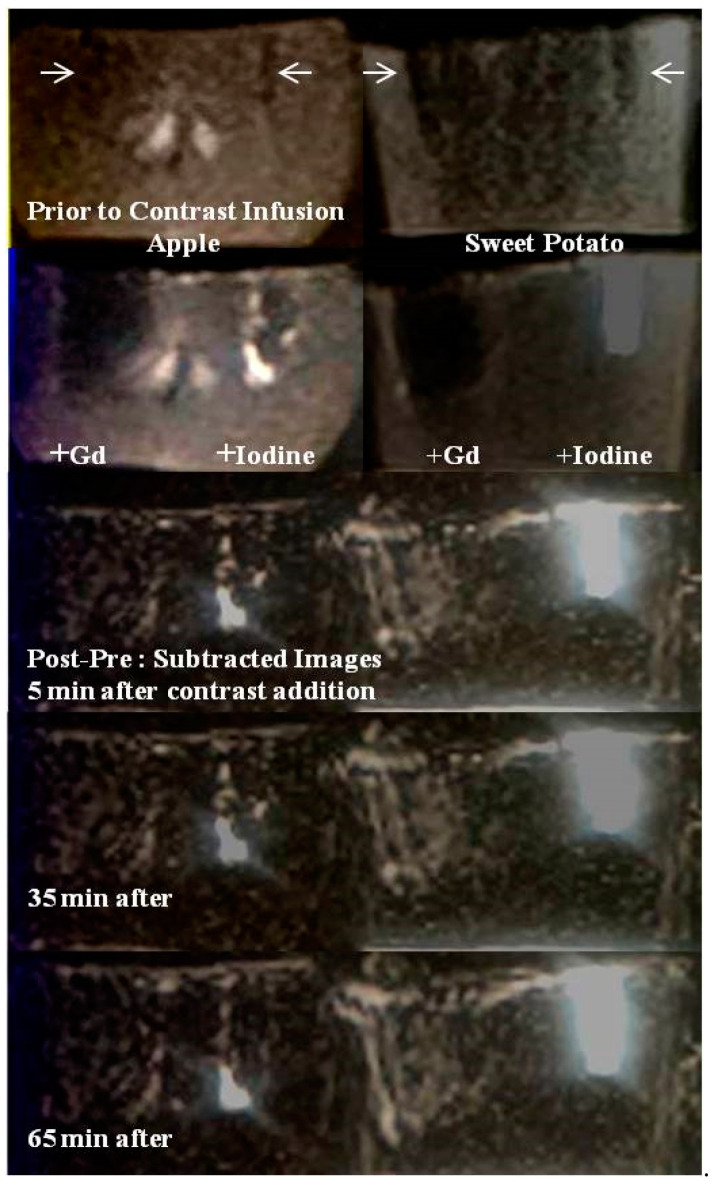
MR images of the mineral-rich model fruits and vegetables. First row: Pre-contrast MR image at 1.5T Siemens system, apple (left) and sweet potato (right). Manually carved wells (2 × 2 × 10 mm^3^ or 0.04 mL volume) are shown (white arrows). Second row: Post-contrast MR image when Gadavist is added at the left wells and Iohexol in the right wells for both fruits. Notice signal loss due to paramagnetic nature of Gd^3+^ and enhanced signal due to Iohexol. Third to fifth rows: Time series subtraction images (post-pre contrast) at time = 5 min, 35 min and 65 min are shown. More pronounced enhancement is seen with Iohexol in sweet potato.
